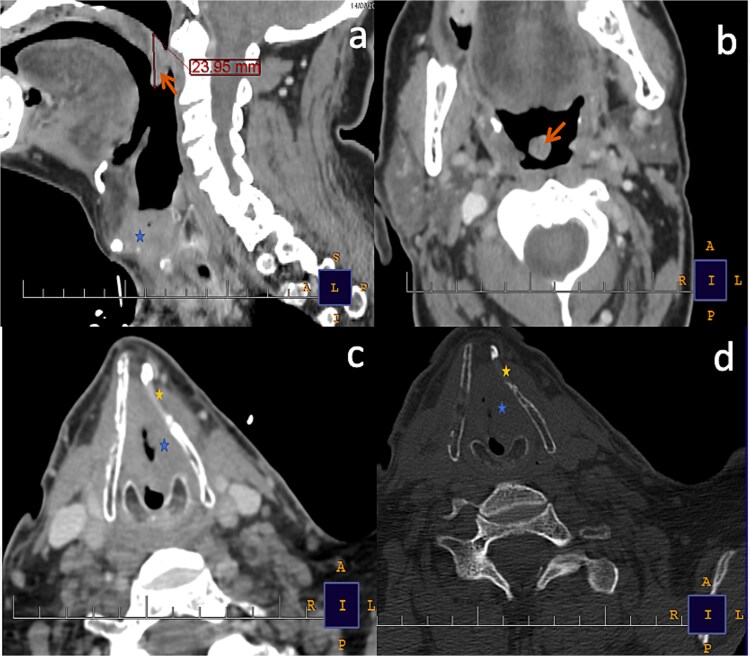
(a) Sagittal and (b) axial contrast-enhanced CT images (soft-tissue window) demonstrate a laryngeal mass centered at the glottic level (blue asterisk), extending to the anterior commissure and causing significant narrowing of the laryngeal lumen. On contrast-enhanced CT, the tumor shows mild heterogeneous enhancement, with attenuation values measuring approximately 65–75 Hounsfield units (HU).The uvula (orange arrow) demonstrates mild, homogeneous contrast enhancement, with attenuation values of approximately 45–50 HU, and measures 23.95 mm in craniocaudal length. (c) Axial contrast-enhanced CT image (soft-tissue window) and (d) axial CT image (bone window) reveal focal lysis of the left thyroid cartilage (yellow asterisk), consistent with tumoral extension from the primary glottic lesion (blue asterisk).
